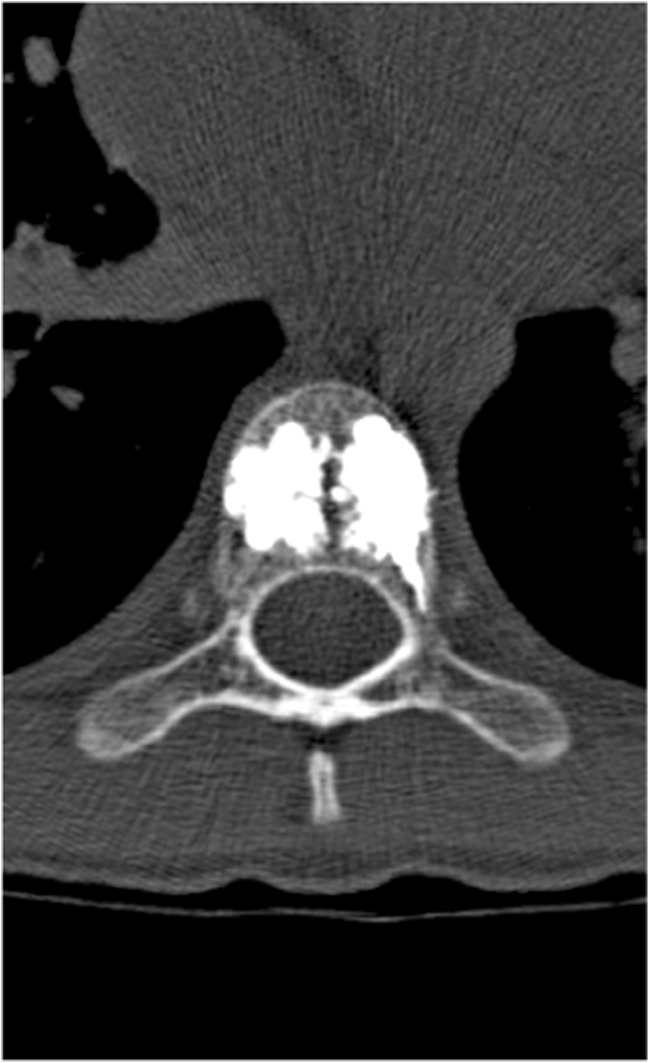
CT control scan of VB (axial)
